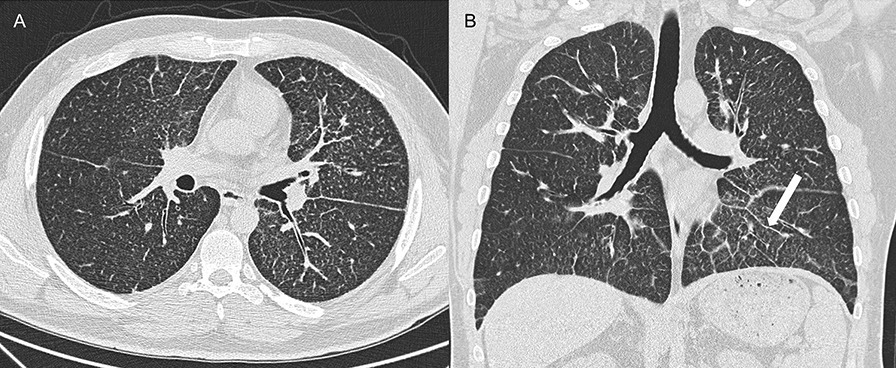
High-resolution computed tomography (HRCT) showing classical findings suggestive of pulmonary alveolar microlithiasis. A. Multiple microcalcifications, axial plane. B. Multiple microcalcifications and septal thickening (arrow), coronal plane
